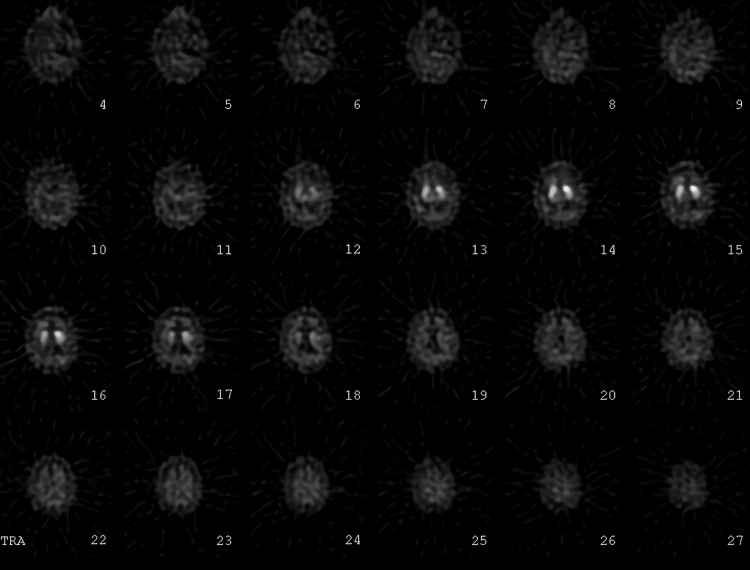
The (123)I-ioflupane SPECT scan with bilateral presynaptic striatal dopaminergic deficits, right worse than left seen in images 13–17 SPECT: single-photon emission computerized tomography
